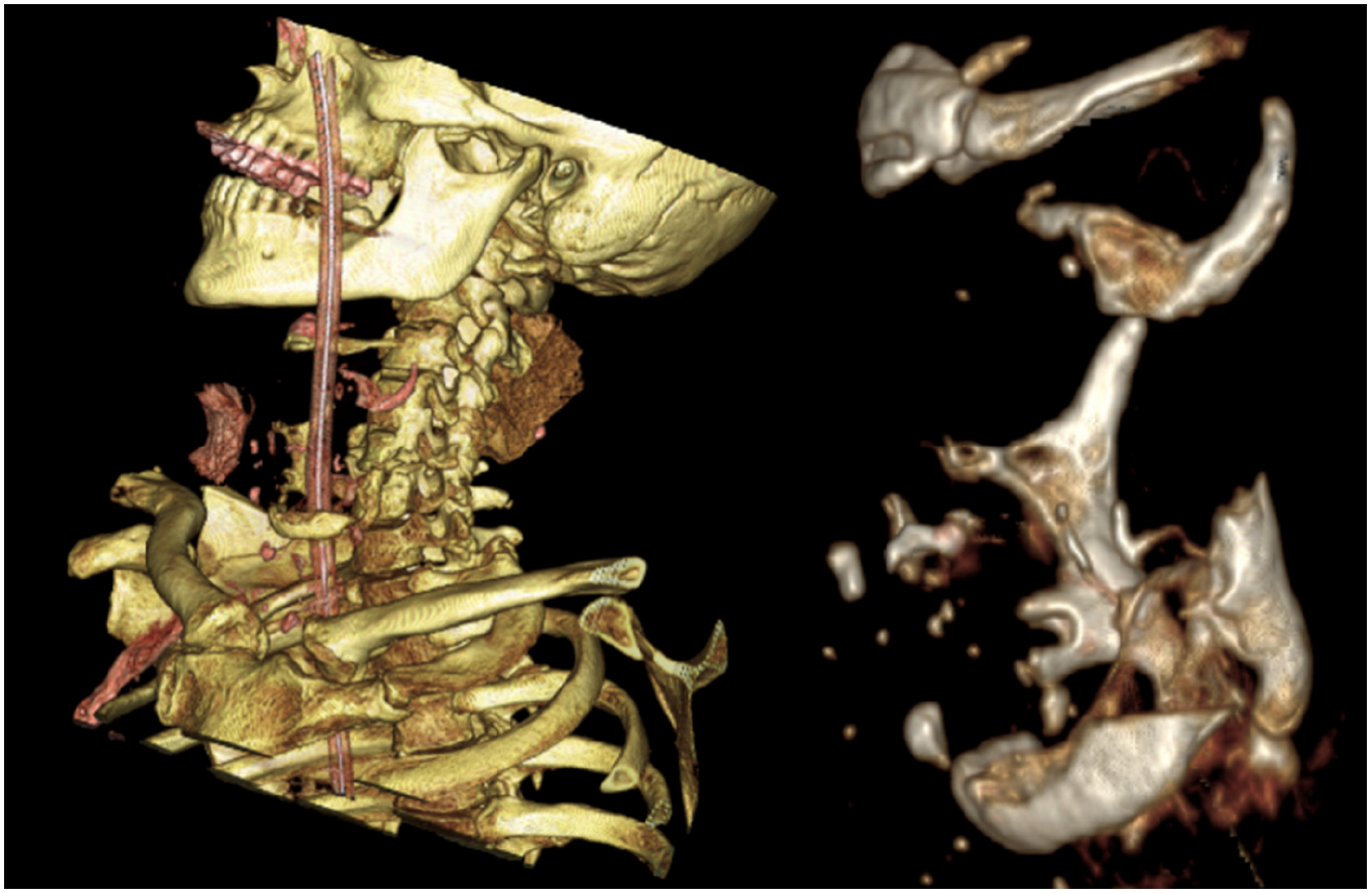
Three‐dimensional CT scan reconstructions of the neck (left) and of the larynx (right), showing a double fracture of thyroid cartilage with an absence of the anterior part of the scutum. The reconstructions were made from the available axial standard images in the PACS system.
